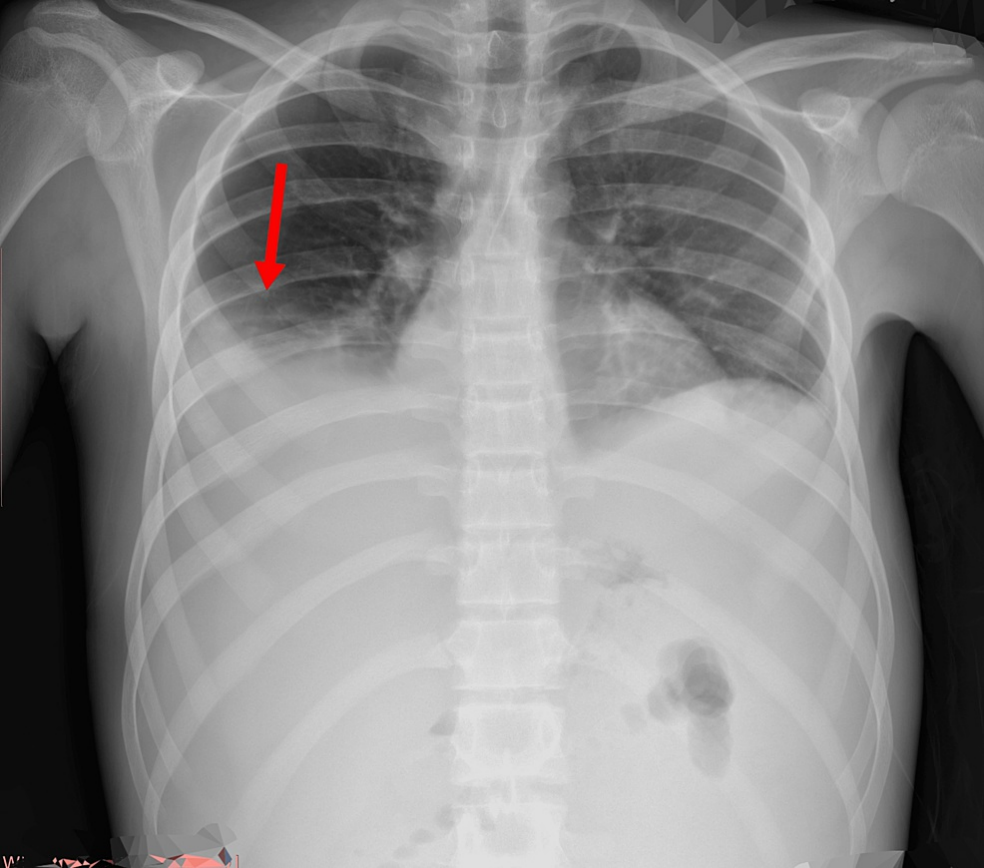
Chest X-ray Blunting of the right side pointed by a red arrow showing pleural effusions.
